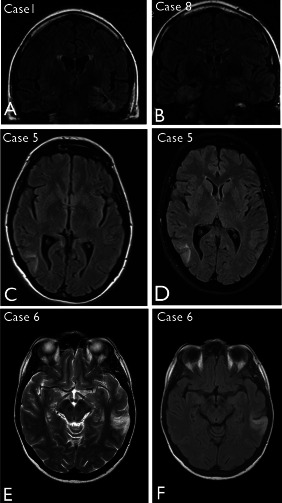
Neuroimaging features of multinodular and vacuolating neuronal tumour. A. Case 1 (as reported in the original case report^45^) showing marked signal change in the left temporal lobe, parahippocampal gyrus and hippocampus on coronal FLAIR but with minimal mass effect; little change was noted over 8 years. B. Case 8. Abnormality was observed in the right mesial temporal region with hyperintensity on coronal FLAIR sequence. C. Case 5. Initial FLAIR MRI sequences at presentation highlighted a lesion in the right posterior temporal lobe as a diffuse cortical abnormality, which did not show significant growth on MRI, as shown in (D) at 21 months following the initial scan. E. Case 6 shown with T2 and (F) FLAIR sequences, highlighting a hyperintense abnormality and the cortical, white matter interface in the left temporal lobe.
